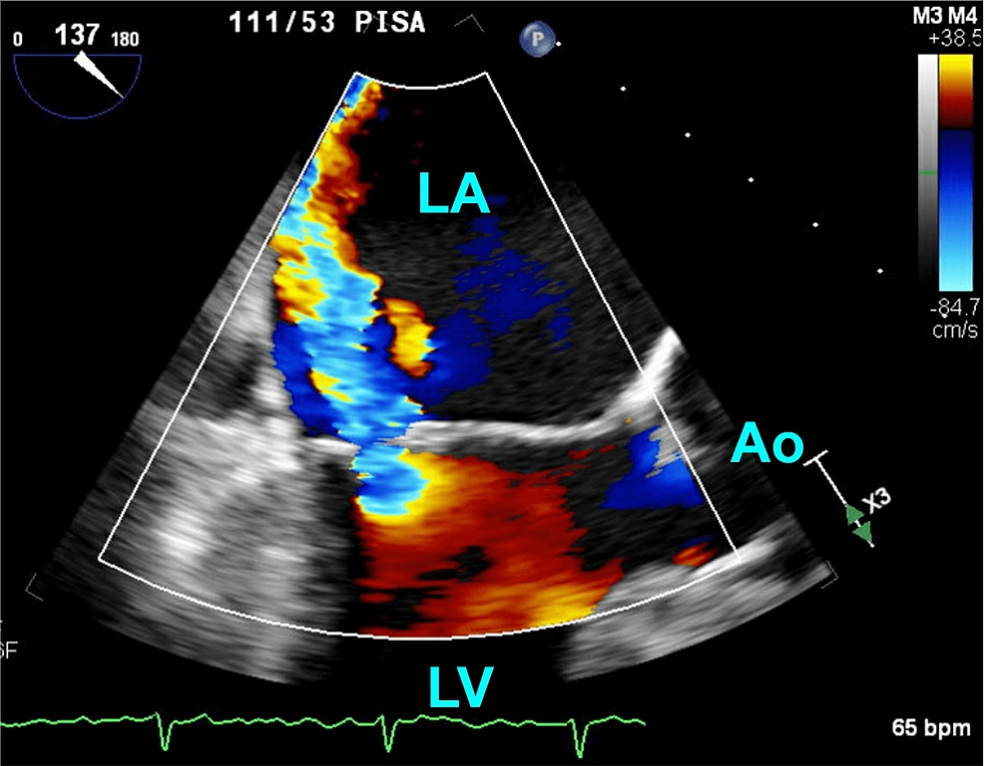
Midesophageal long view of the transesophageal echocardiogram at 10 months after Watchman placement. This was performed as part of the pre-operative evaluation for potential mitral valve repair. Color doppler showed a high-velocity, posteriorly directed mitral regurgitant jet ejecting retrogradely from the left ventricle. LV: left ventricle, LA: left atrium, Ao: aorta
